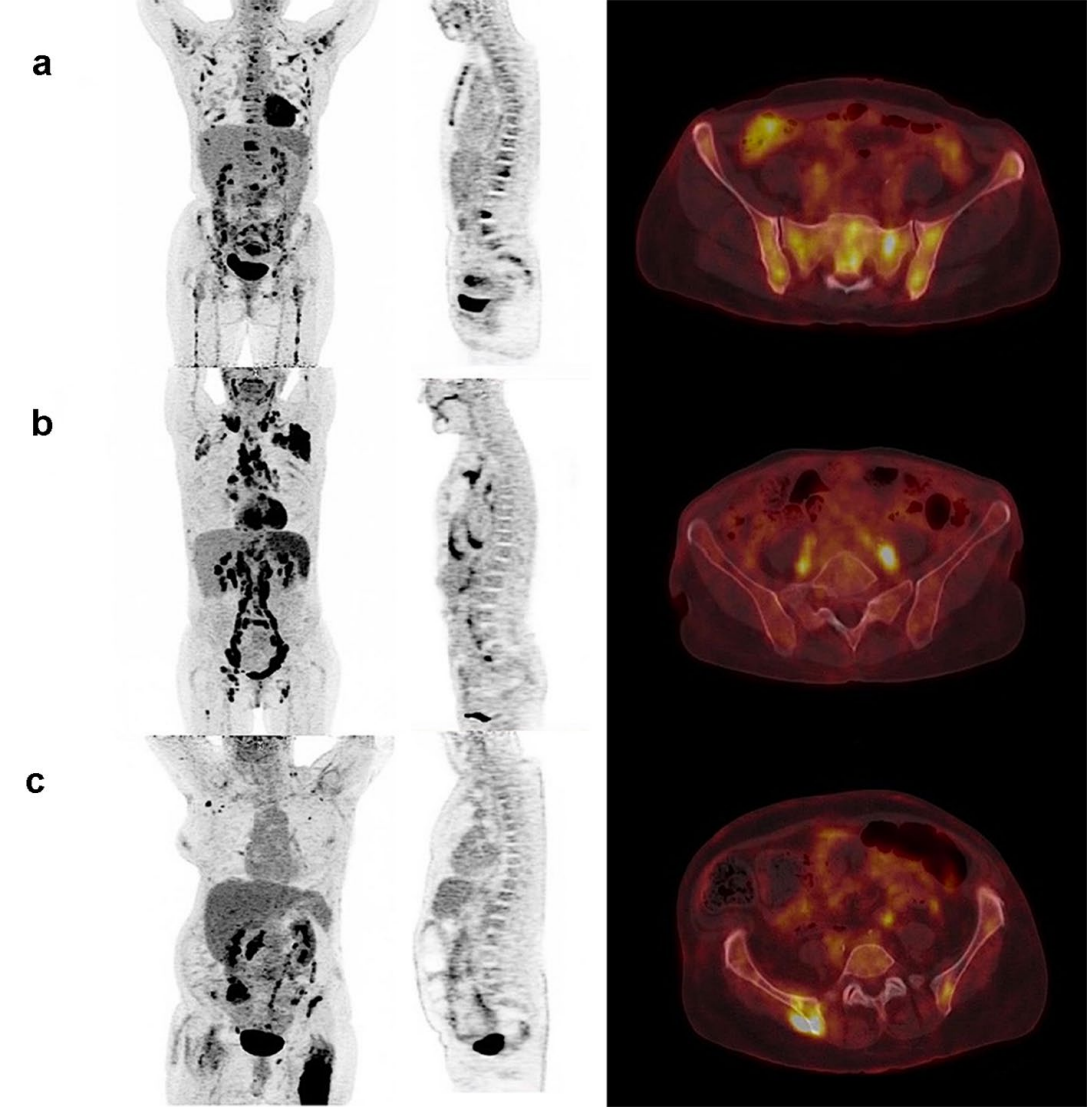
Representative MIP PET, sagittal PET and axial PET/CT images of patients PET +/BMB + (a); PET −/BMB + (b) and PET +/BMB − (c).
